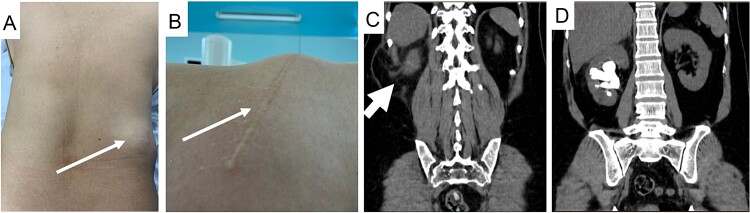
Images of the patient with right flank incision and hernia bulge with clinical picture (A and B, thin arrow), hernia sac on CT scan (C, thick arrow), and staghorn stone (D).
